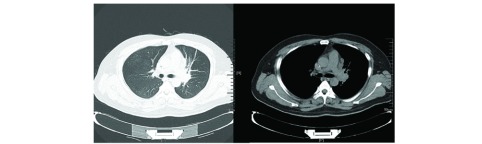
胸部CT（2016-12-14）：左肺门软组织影，大小约4.4 cm×2.6 cm，分叶状，密度尚均匀，周围可见肿大淋巴结影。 Chest CT scan (2016-11-12): A lobulated soft tissue giant tumor located in the left pulmonary hilum with the size of about 4.4 cm×2.6 cm showed homogeneous density; lymphadenopathy around it can be seen.
